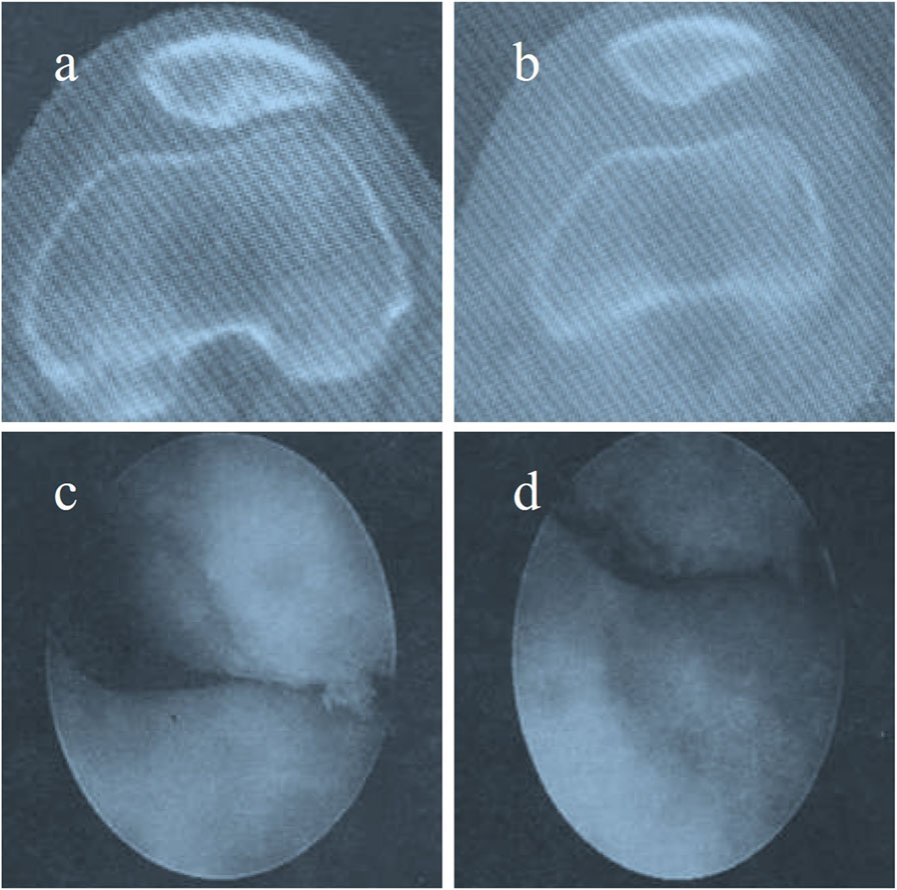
Comparison of preoperative and postoperative radiographic data of CT scan and arthroscopic examination of the ELPS patient. a Preoperative CT scan image revealed lateral articular space stenosis. b Postoperative CT scan image revealed that the lateral joint space returned to normal. c Preoperative arthroscopic examination revealed lateral articular space stenosis. d Postoperative arthroscopic examination revealed that the lateral joint space returned to normal
